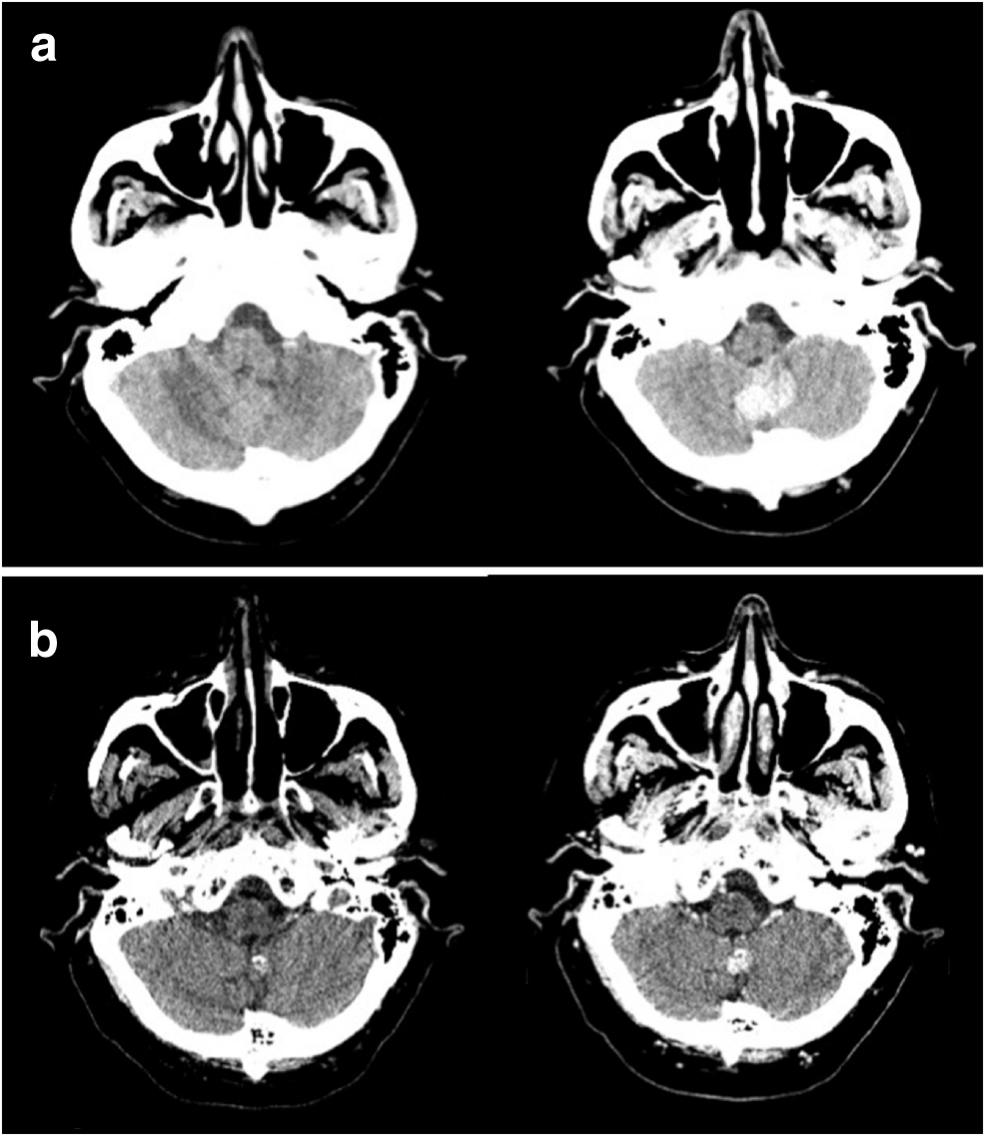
Post-RT CBM. Computed tomography, axial plane. a Pre- and post-contrast CT scan of a patient with a primary adenocarcinoma of the lung shows an inferior vermian metastatic lesion with intense enhancement and slight mass effect. b Follow-up CT scan of the same patient after stereotactic radiosurgery of the vermian lesion shows the appearance of a small nodular calcification within the lesion in the unenhanced CT, together with a definite reduction of the volume and of the associated mass effect
